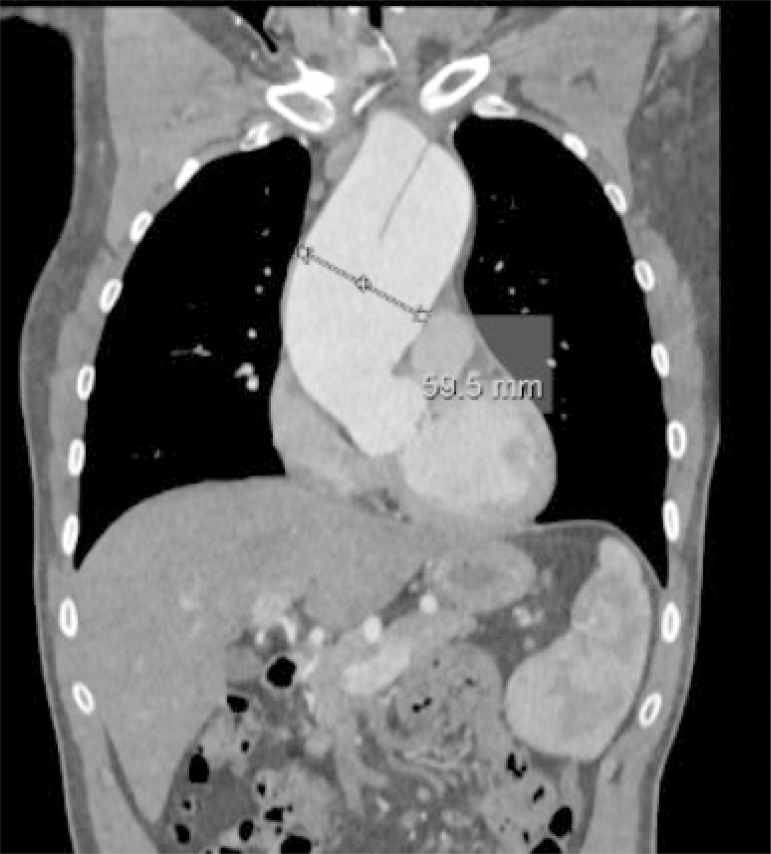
Thoraco-abdominal CT scan on the day of the first diagnosis, showing aortic aneurysm and dissection membrane.
